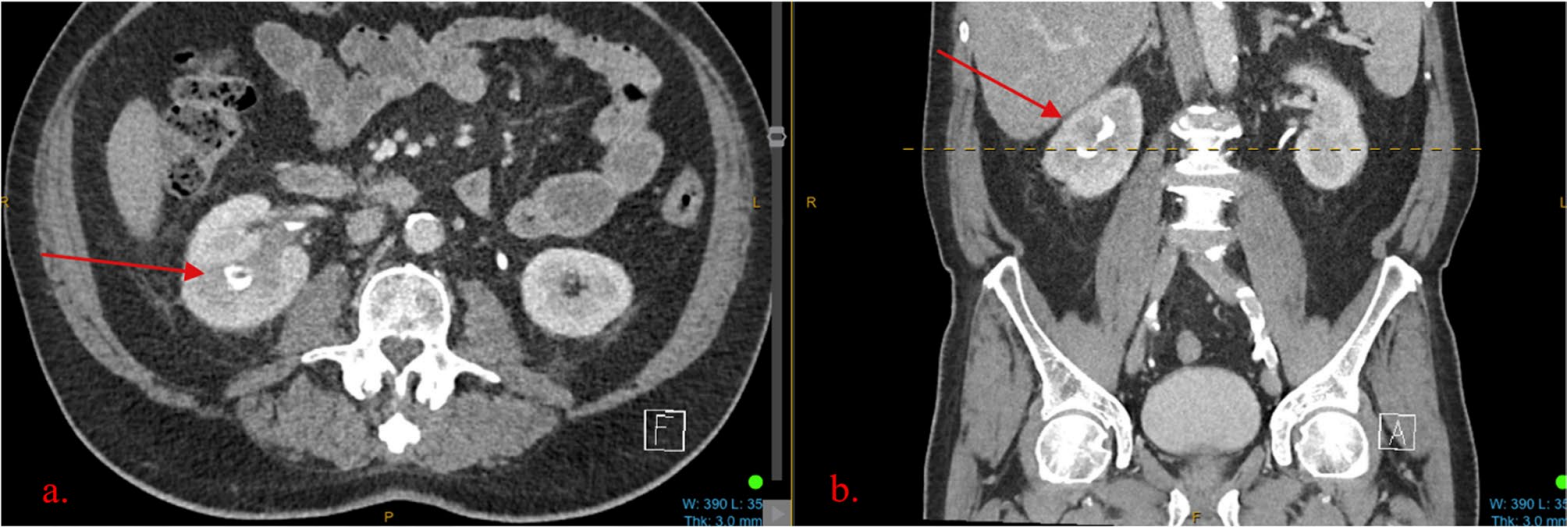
CT Intravenous pyelogram. a An axial view demonstrating renal papillary necrosis at the right upper pole with underfilling, associated mass effect at the renal pelvis. b A coronal view demonstrating the same
